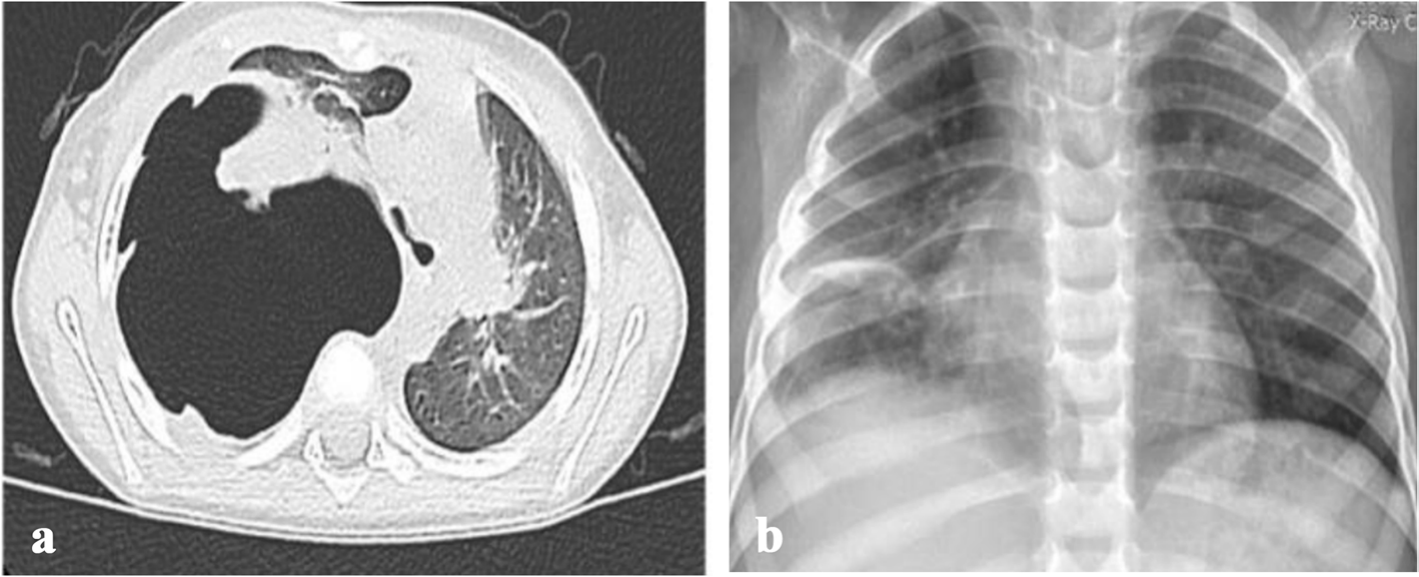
3-year-old child presented with a) extensive necrotising pneumonia. Follow up X-ray at 1 year showed marked improvement
